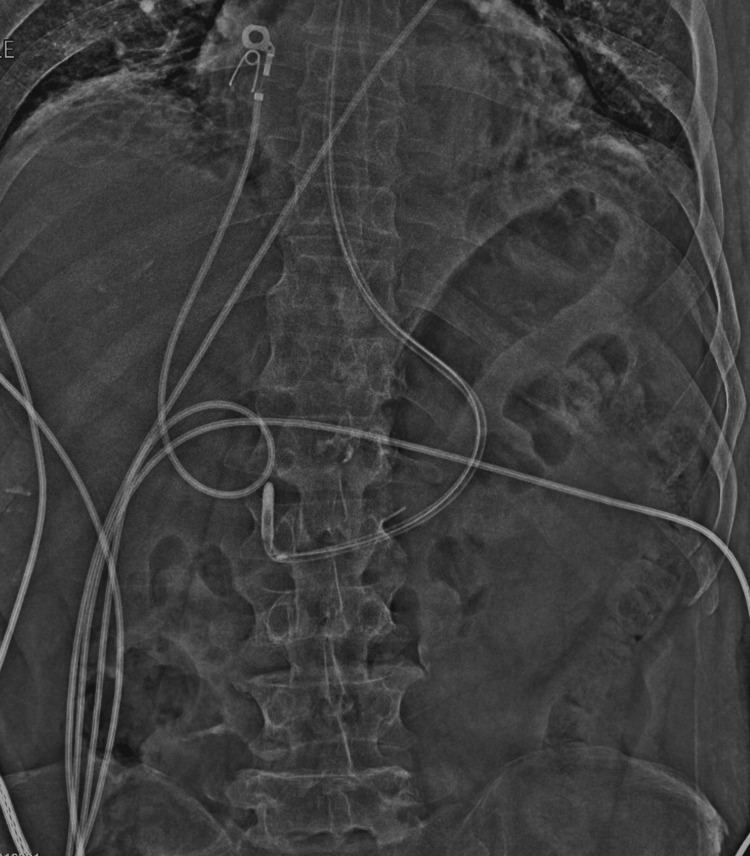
Abdominal radiograph confirming intragastric positioning of the small-bore nasogastric feeding tube (Corpak) with guidance stylet.
